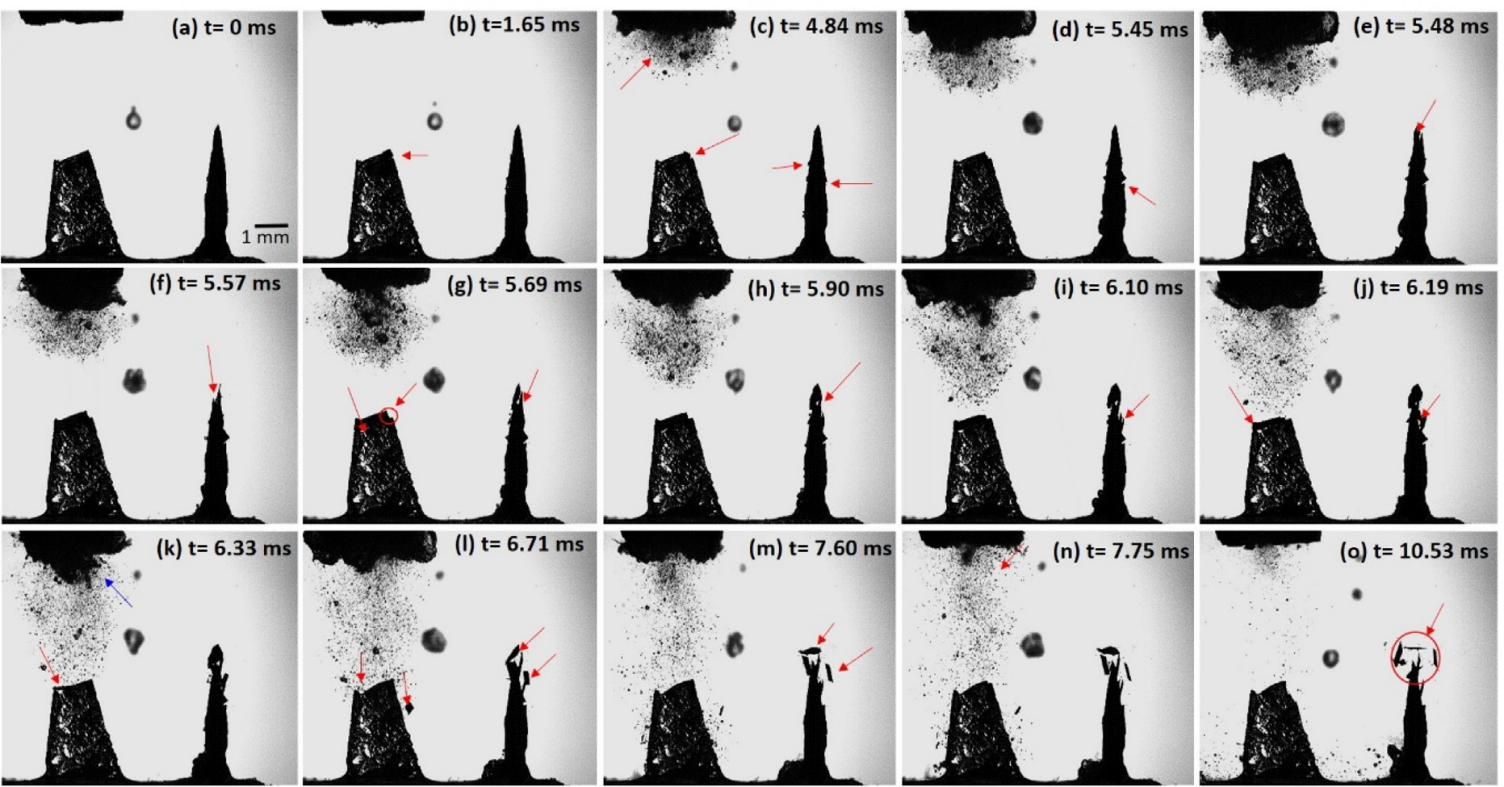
High-speed images of fragmentation of primary Al_3_Zr crystals captured at 100 kfps when subjected to ultrasonic cavitation. The supplementary video can be accessed with the online version of the article as video 4.
